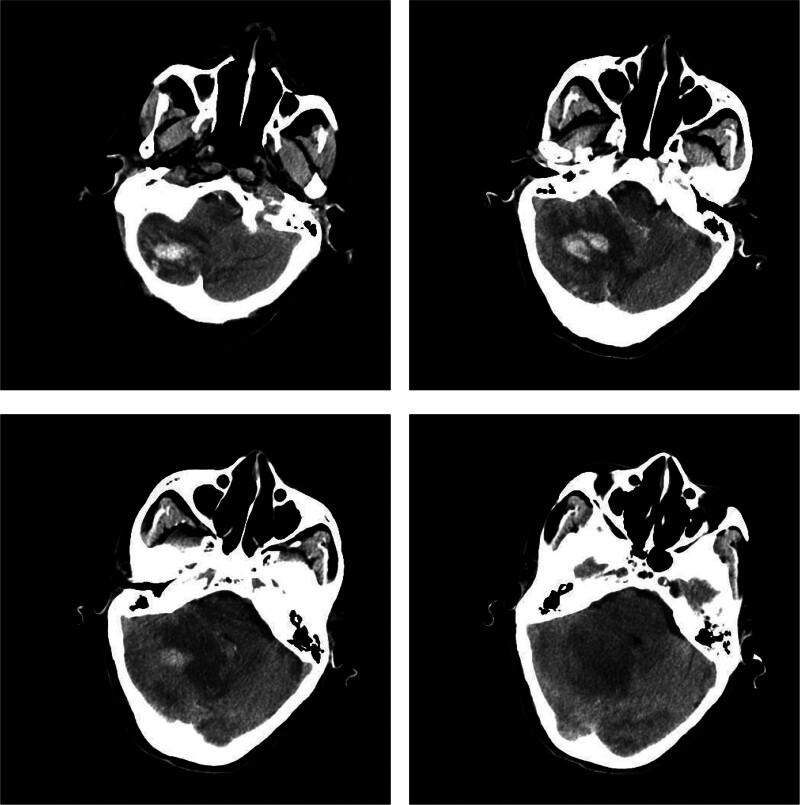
Review of head CT image before craniotomy for exacerbation on the day of admission. CT = computed tomography.
